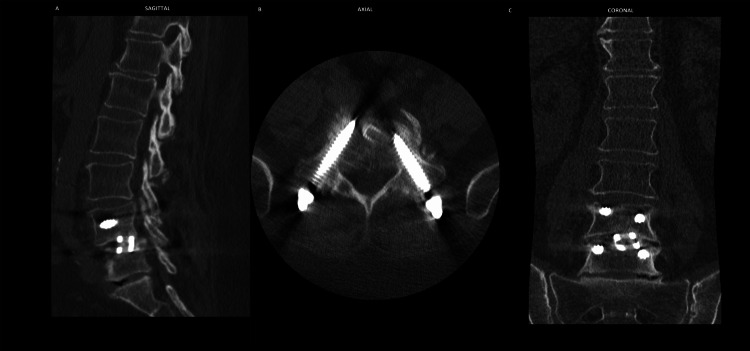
Lumbar CT five months postoperatively showing hardware condition and placement from three views (sagittal, axial, and coronal) The sagittal view (A) demonstrates placement in relation to spondylolisthesis, the axial view (B) shows alignment of the fixed pedicle screws, and the coronal view (C) displays screws and interbody in relation to each other.
